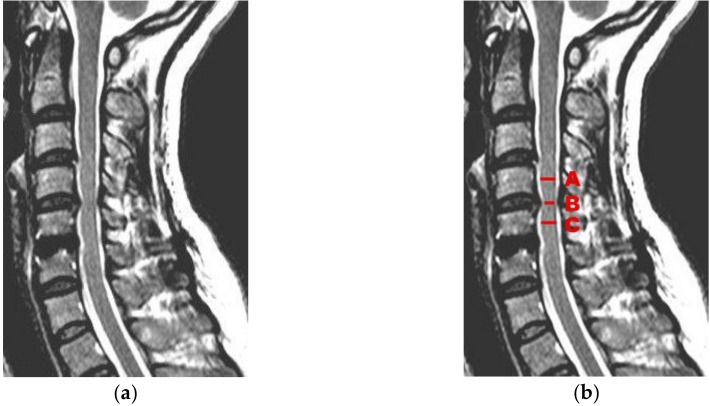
(a) MRI of the cervical region with well-known fusion of cervical vertebrae 5 and 6 and new constriction of the spinal cord between vertebrae 4 and 5; (b) the same shown with three diameters, A, B, and C. The value “Relative Spinal Cord Diameter” (RSD):= 2B/(A+C) can be used in the adapted domain for quantification of the constriction.
